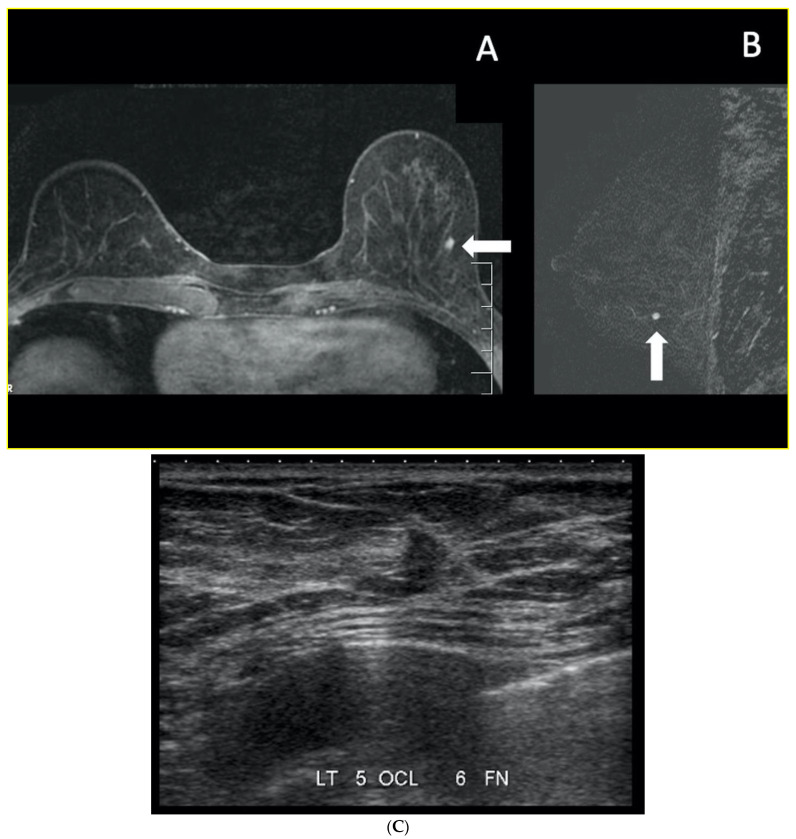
Courtesy of Dr. Anabel Scaranelo. High-risk screening with breast MRI in a 52-year-old woman with a family history of breast cancer in her sister. Screening mammography (not shown) was negative, with category C density. Focus of enhancement (arrow) in the lower outer quadrant of the left breast, (A) axial and (B) sagittal T1-weighted 3D-gradient echo with fat saturation 2nd minute post contrast. (C) The patient was recalled for a second look ultrasound that showed correlation with a suspicious subcentimeter mass lesion at 5 o’clock (arrows). An ultrasound-guided core needle showed grade 2 invasive ductal carcinoma.
